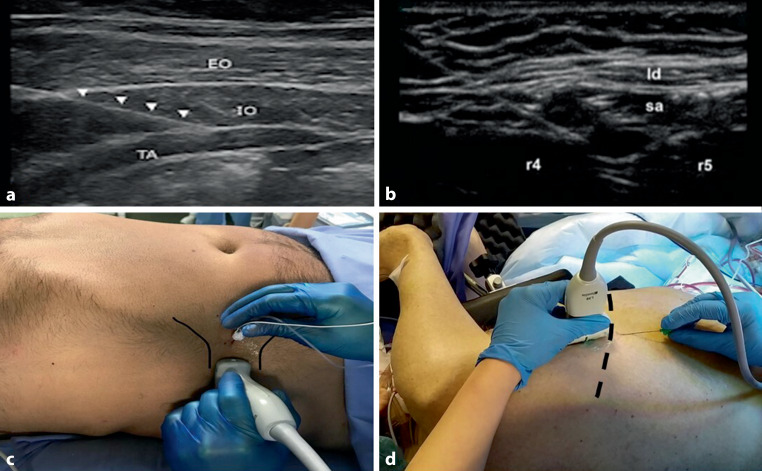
Pre-emptive locoregional anesthesia using ultrasound-guided transversus abdominal plane (TAP) block (a–c), and serratus anterior plane (SAP) block (d). *EO* External oblique, *IO* internal oblique, *TA* Transversus Abdominis
